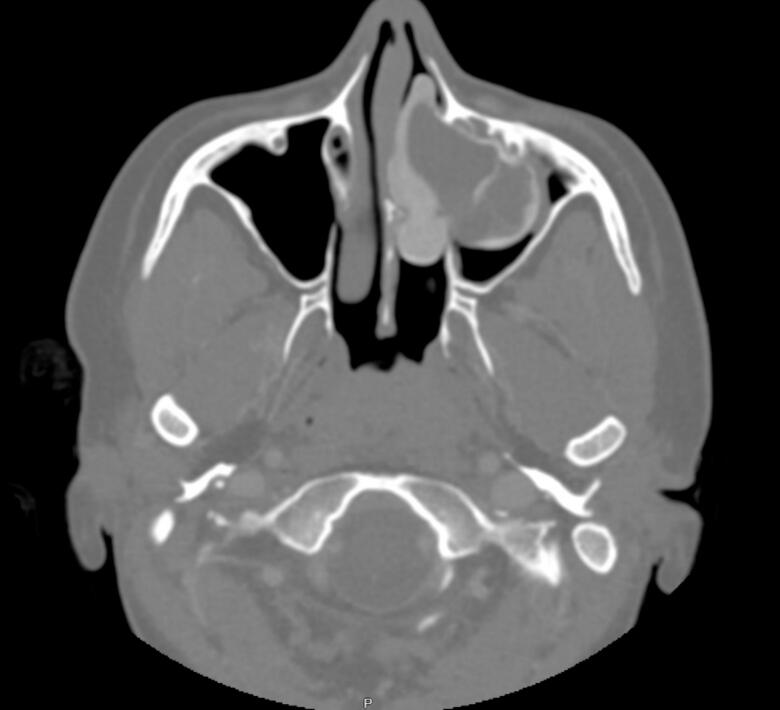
Axial view Computed Tomography (CT) of paranasal sinuses showing left sided nasal cavity lesion with peripheral calcification extending to left maxillary sinus.
